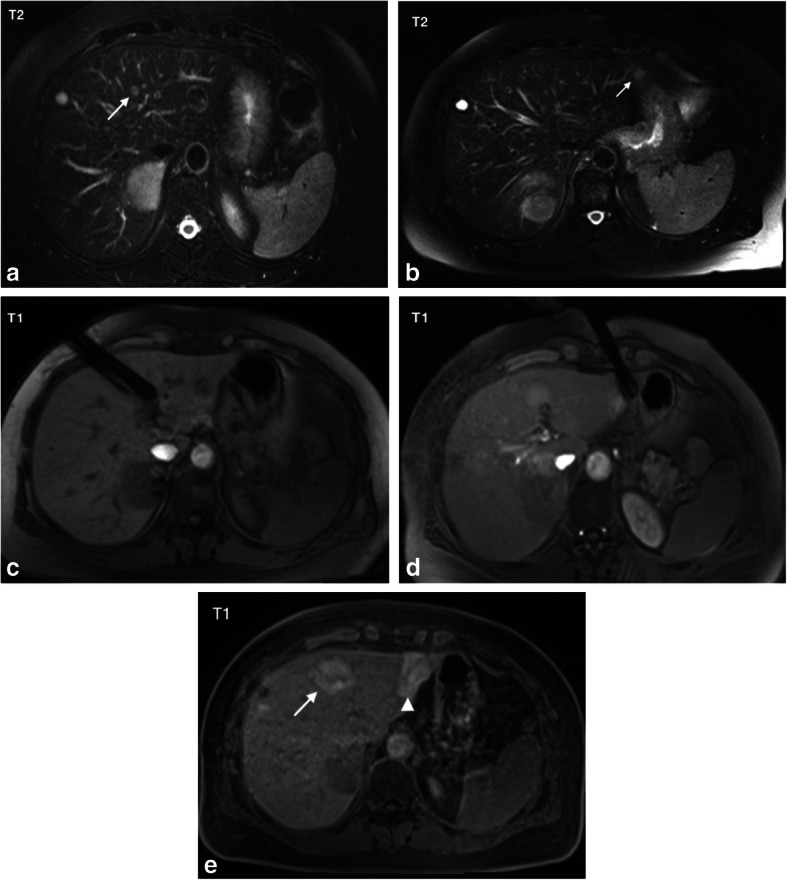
62-year old female patient with hepatic metastases from a uveal melanoma in both liver lobes. According to tumor-board decision, right hemihepatectomy was planned after thermoablation of a metastasis in the left liver lobe. Pre-interventional T2-weighted TSE imaging (a) shows the target lesion planned for thermoablation. However, intra-procedural planning imaging (b) reveals a new small lesion subcapsular in segment 2 (arrow). Both tumors could be treated during the same procedure as depicted by intra-interventional T1-weighted sequences (c and d) showing two radiofrequency antennas targeting both target tumors. T1-weighted control imaging shows the T1 hyperintense ablation zones covering both target tumors (e). Right hemihepatectomy was conducted 1 month after ablation
